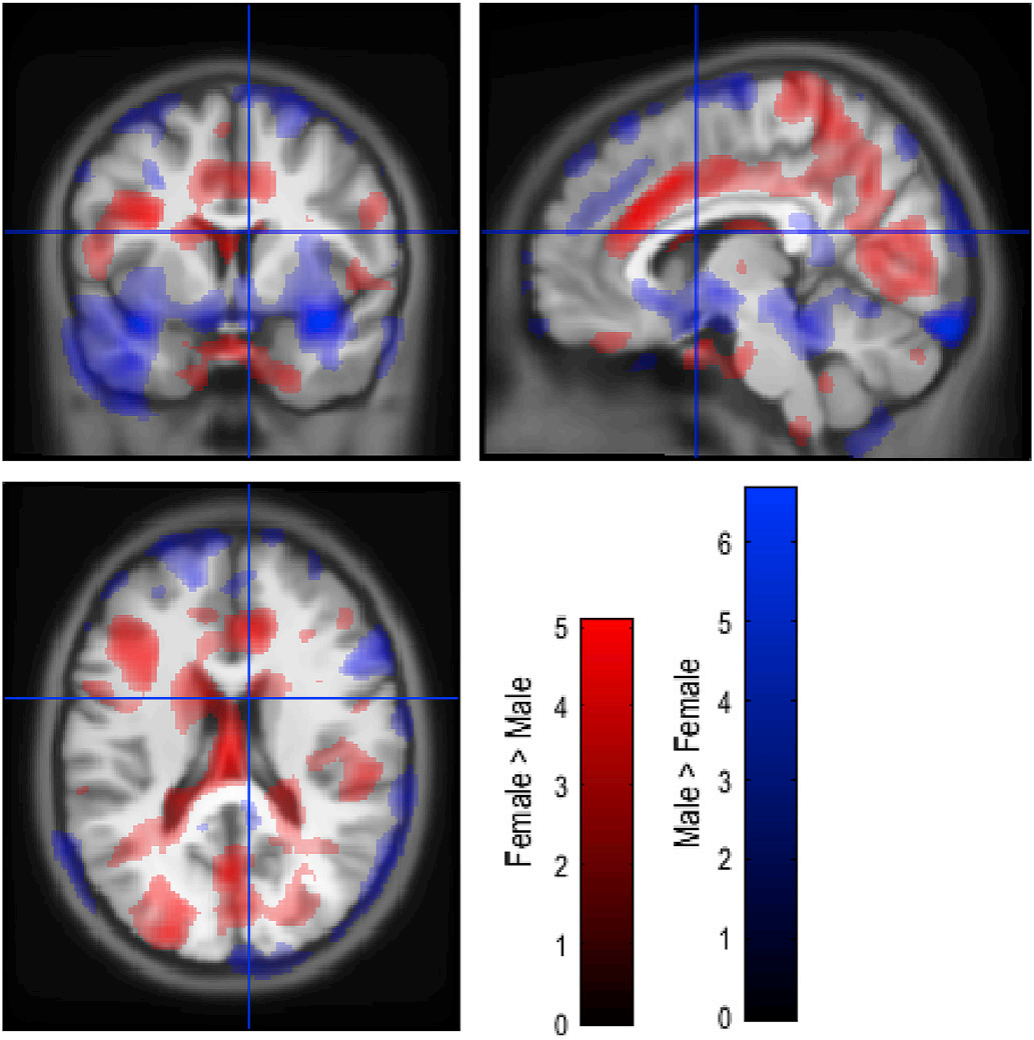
Regions showing sex differences in GM volume. Red shows regions with greater GM volume in females. Blue shows regions with greater GM volume in males. T-statistic maps thresholded at p ​< ​0.05 uncorrected were overlaid on the average of individual T1-weighted images warped together into MNI space.
